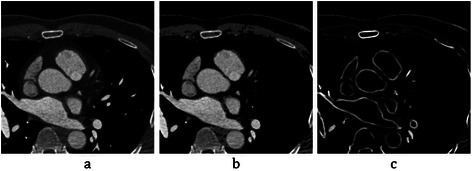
Coronary artery enhancement. (a) Selected slice of the CTA. (b) Apply the mask. (c) The result of enhancement algorithm.
